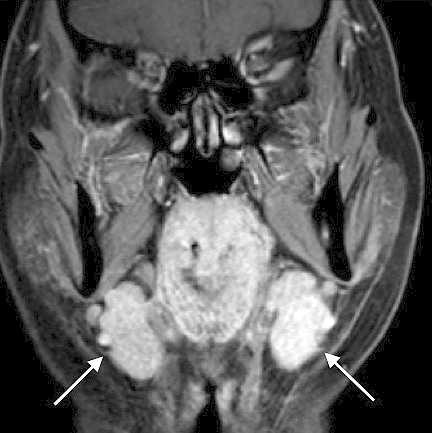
MRI findings of IgG4-related salivary gland lesions exhibiting bilateral swelling (arrows).
